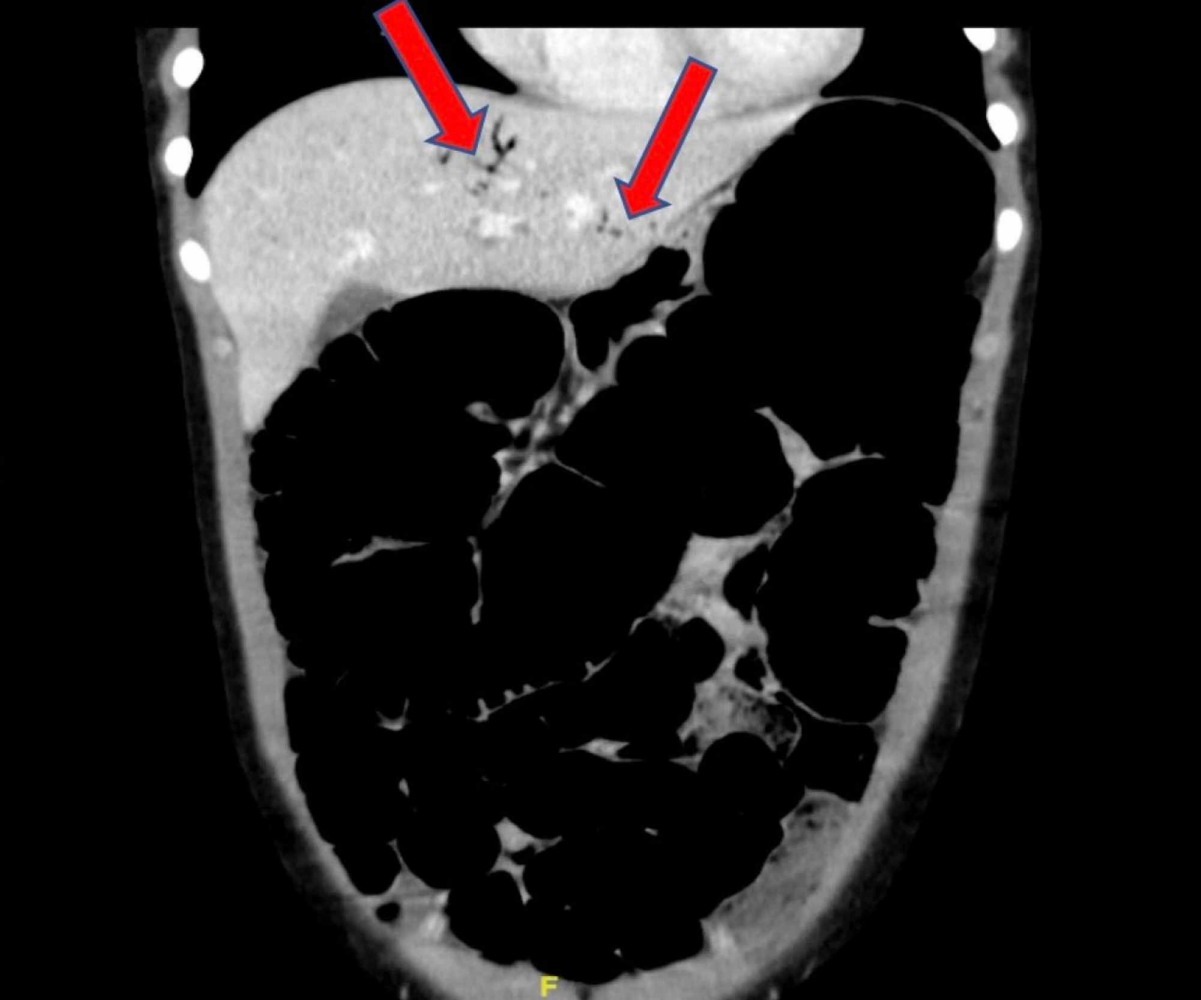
Coronal view CT scan of the abdomen showing marked hepatic venous portal gas
